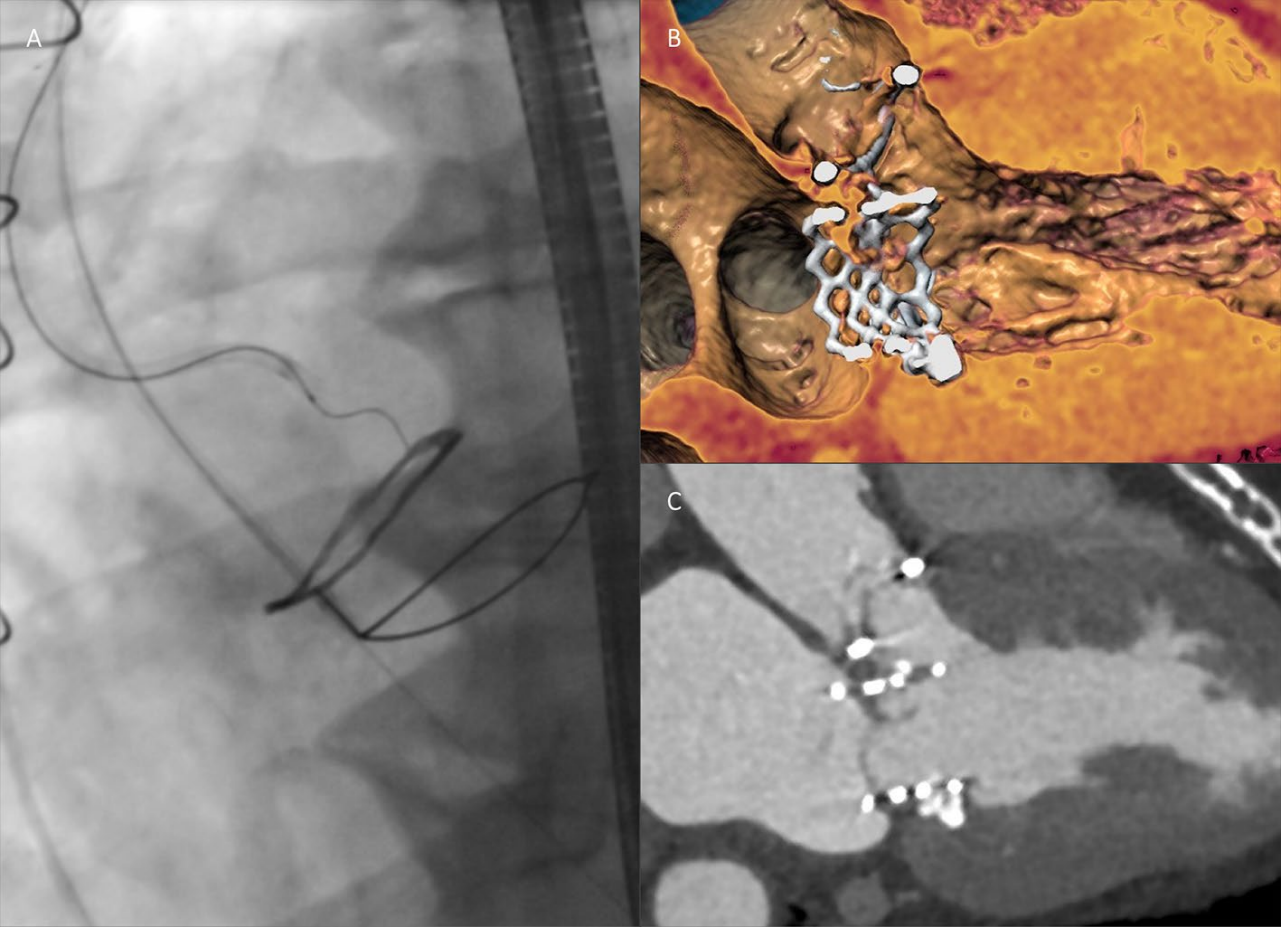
BASILICA failure. A Impenetrable calcified leaflet (Sorin Mitroflow surgical valve). B Wire-interference with a THV in mitral position (Sapien-in-MAC), Sorin Mitroflow aortic valve, pre-TAVI three-dimensional CT reconstruction. C pre-TAVI CT (see B). *BASILICA* bioprosthetic or native aortic scallop intentional laceration to prevent iatrogenic coronary artery obstruction, *CT* computed tomography, *MAC* mitral annulus calcification, *TAVI* transcatheter aortic valve implantation, *THV* transcatheter heart valve
